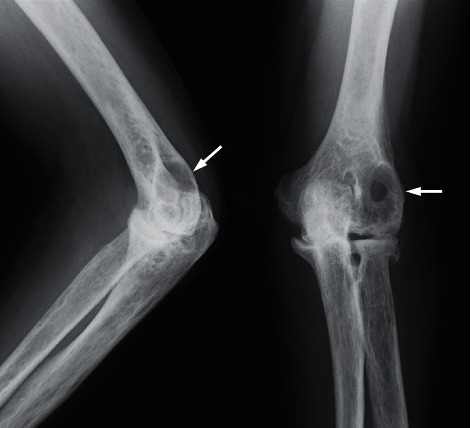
Plain radiograph of the left elbow at one month after administration of denosumab every three months. The transparent area showed little improvement effect compared to Figure 5 (arrow).
